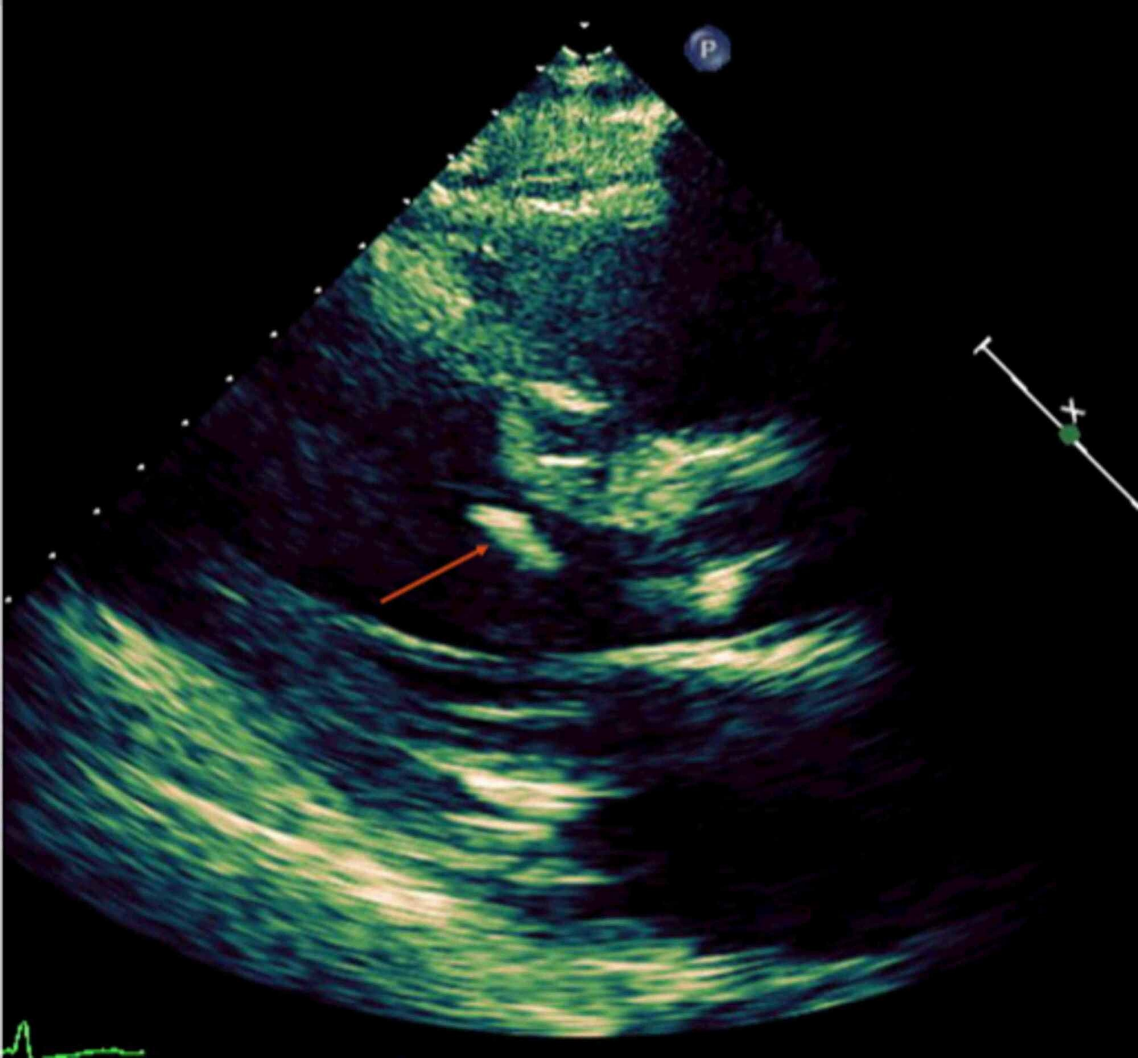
Transthoracic echocardiogram showing mobile hyperechoic echodensity (red arrow) near the basal septum of the left ventricle
